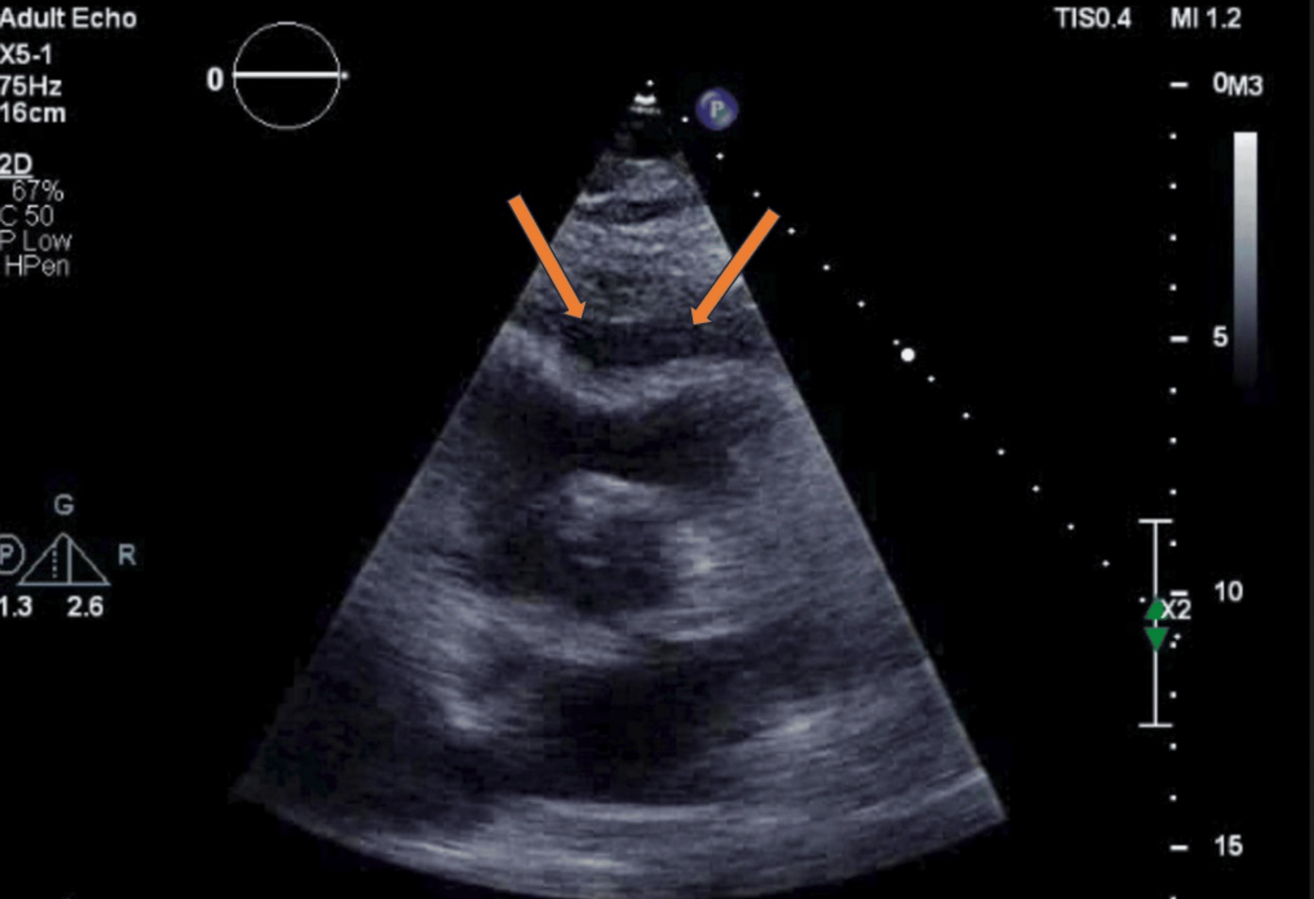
2D echocardiogram demonstrating early right ventricular diastolic collapse, consistent with early cardiac tamponade physiology.
